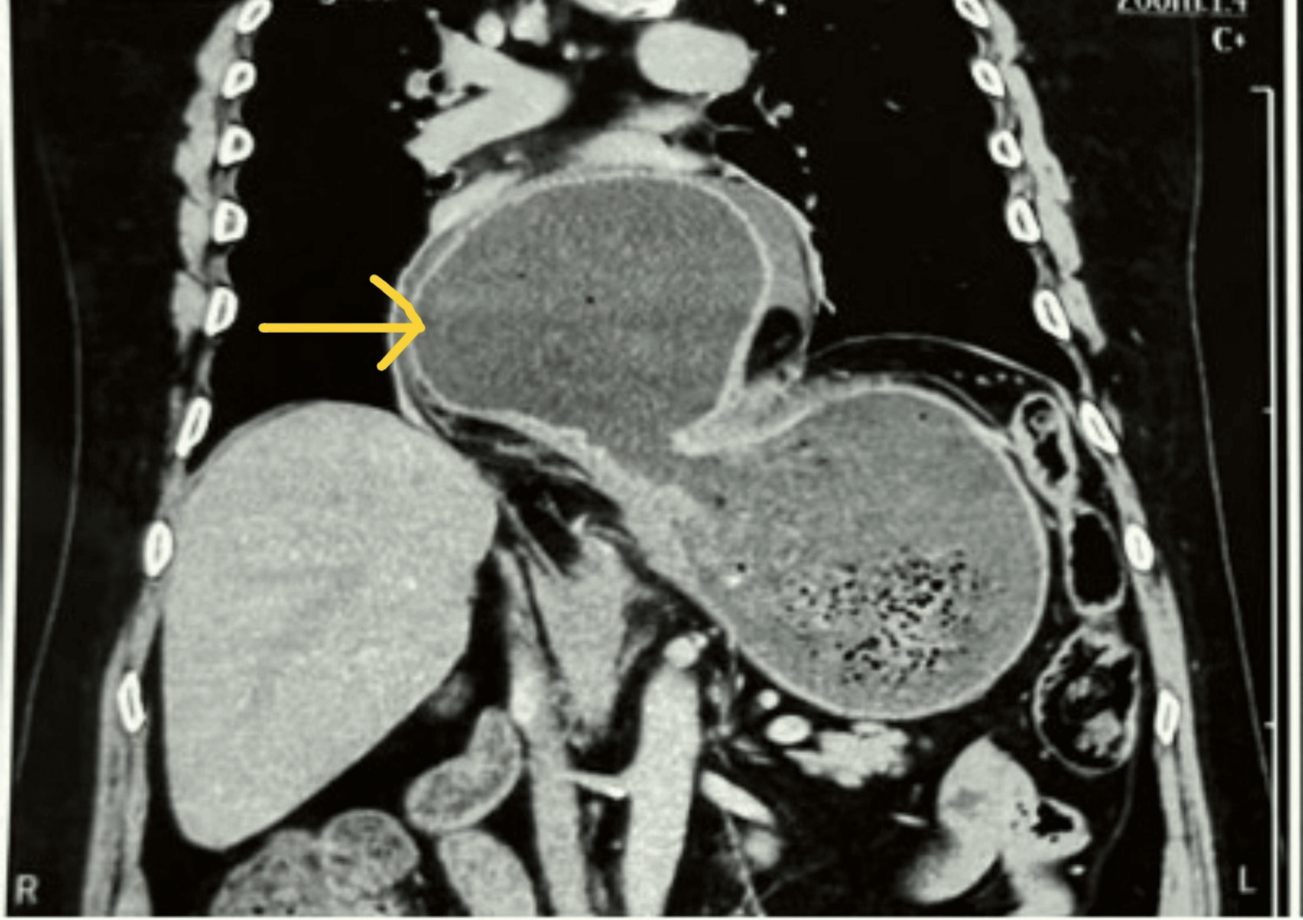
CECT showing herniated stomach in the thoracic cavity CECT: contrast-enhanced computed tomography
